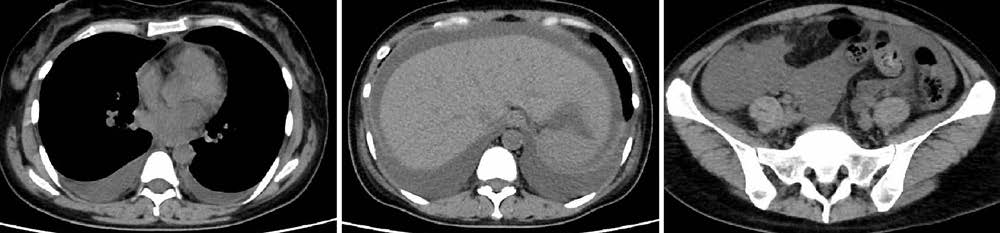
Abdominal computed tomography image on admission. Presence of pleural and ascitic effusion.
